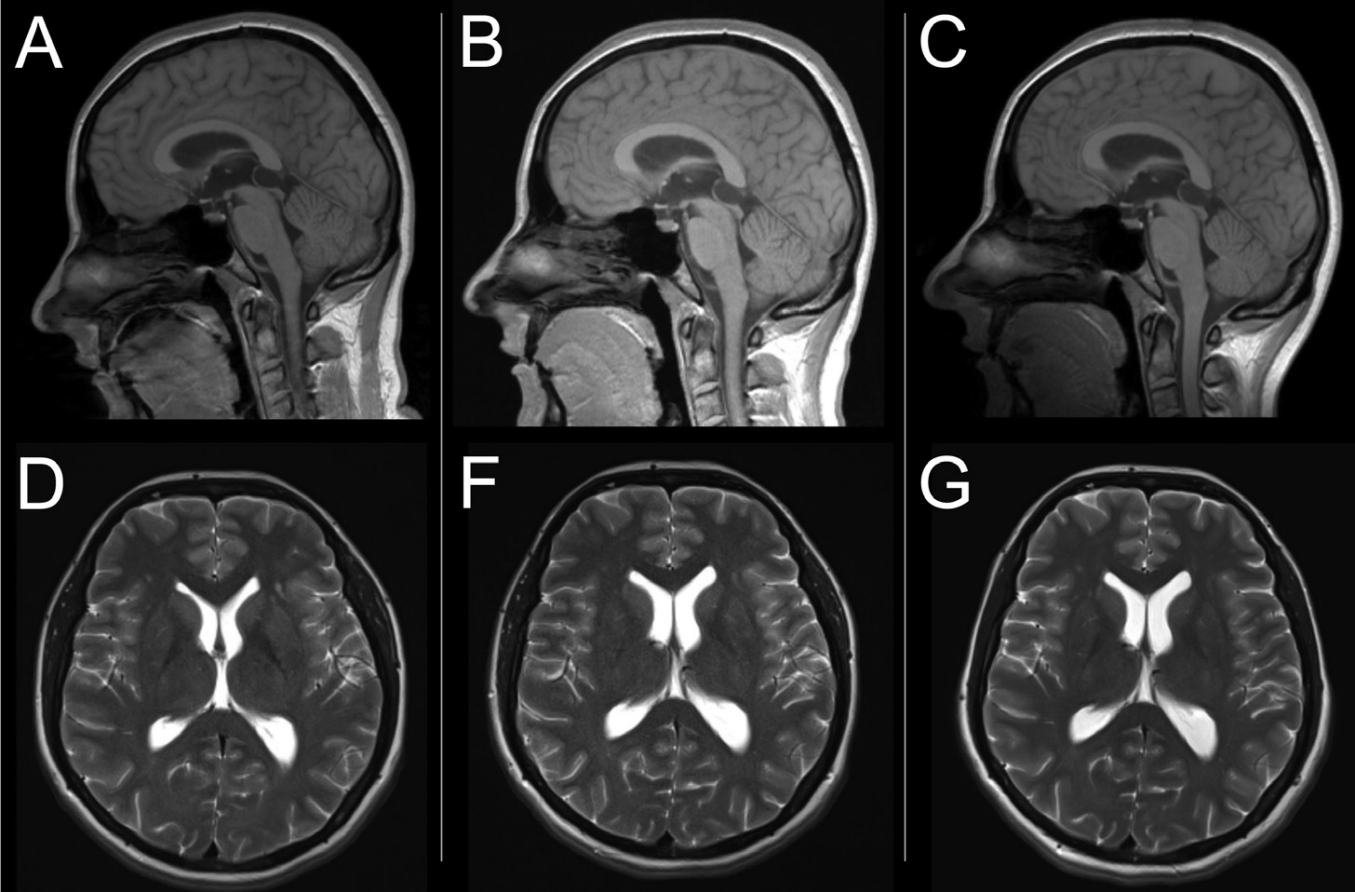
Sagittal T1 slices at the first (A), second (B), and third (C) magnetic resonance imaging (MRI) exam showed a large pineal cyst with compression of quadrigeminal plate and near-obstruction stenosis of the aqueduct of Sylvius. Axial T2 slices at the level of lateral ventricles at the first (D), second (F), and third (G) MRI exam showed a minimal enlargement of ventricular system between the first and second MRI exam.
